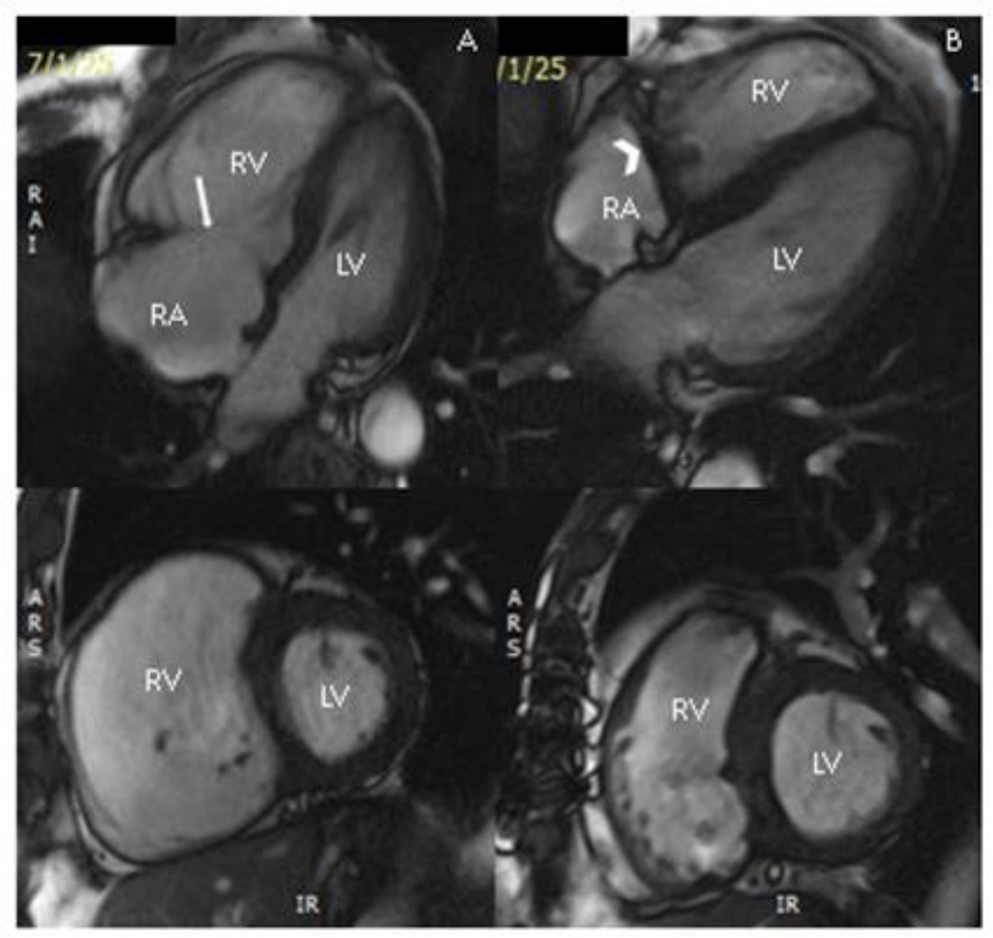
Cardiac magnetic resonance images before surgery (A) demonstrating severe right ventricular dilatation and flattening of the inter-ventricular septum, and post-tricuspid valve replacement (B) demonstrating significant reduction in right ventricular volume; top panel: still from four-chamber cine sequence; lower panel: still from short-axis cine stack at mid-ventricular level. RV, right ventricle; LV, left ventricle; RA, right atrium; arrow, native tricuspid valve; chevron, tricuspid valve replacement.
